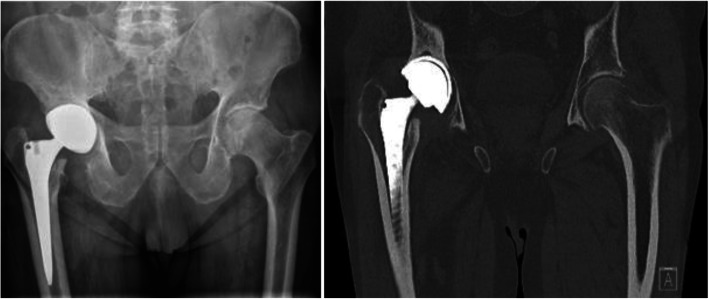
A-P X-Ray and CT-Scans showed the loosening of the prosthesis
